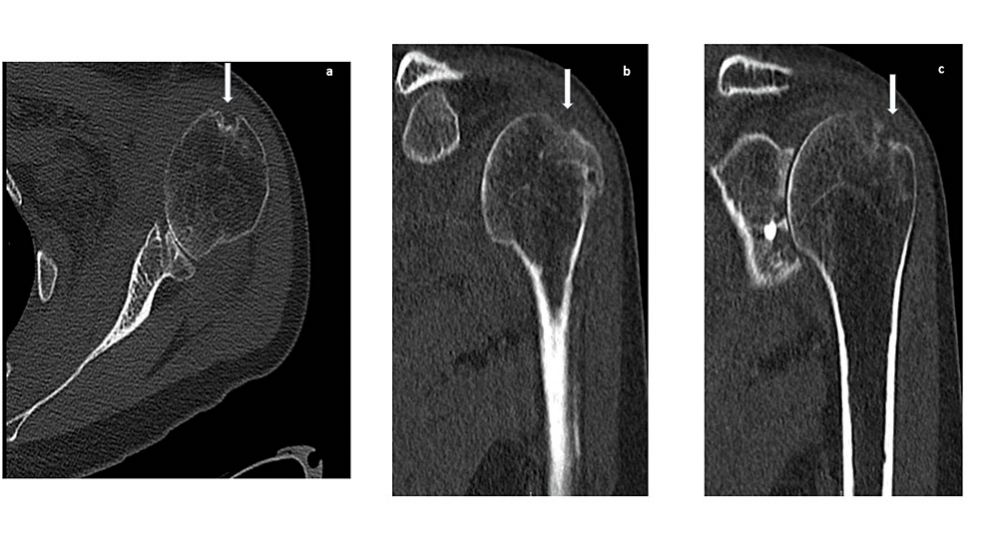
Shoulder computed tomography in axial view (a) and coronal view (b, c) after the removal of the screw. In all panels, osseous erosion and destruction of the cortical bone of the humeral head are present (arrows).
